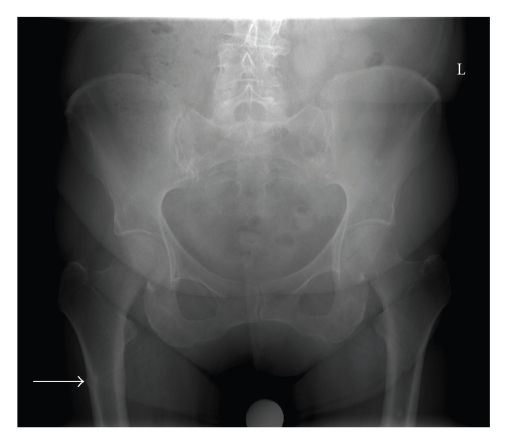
X-ray taken at first presentation of thigh pain on the right side. There is a sign of discrete lateral cortex thickening.
